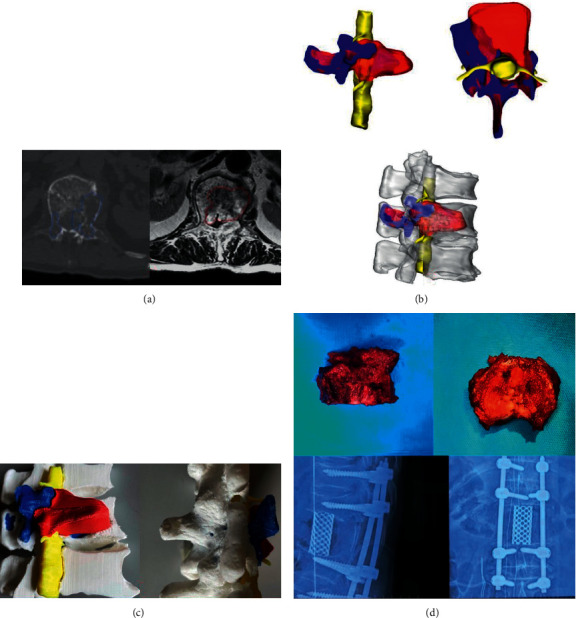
(a) The extent of bone destruction and bone tumor invasion at each level was captured by CT and MRI. (b) The reconstructed 3D images were fused to show the anatomical relationships. The spinal cord was shown in yellow. The extent of tumor invasion was shown in red. The extent of bone destruction was shown in blue. (c) 3D images were printed into the PLA model. (d) Gross view of the tumor resected and postoperative X-rays.
